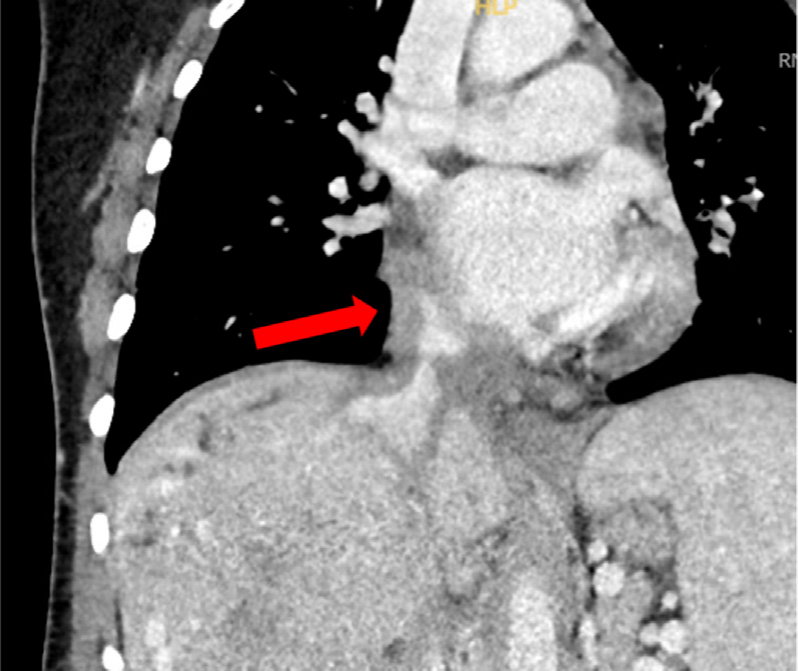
Coronal View of the Computed Tomography Angiography of Chest Infiltrative mass extending from the IVC into the RA. Abbreviations as in Figure 1.
